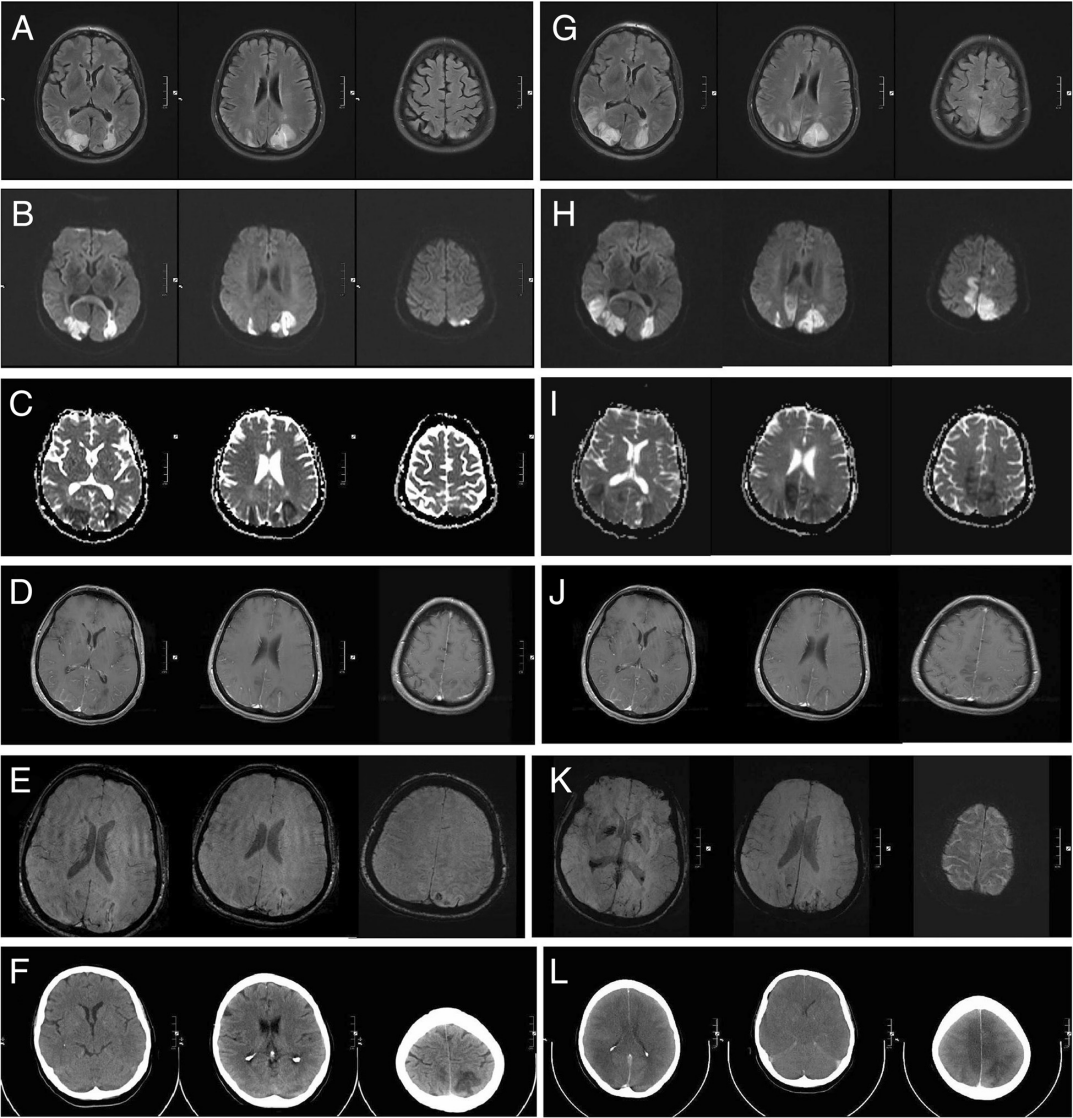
Serial magnetic resonance imaging (3 Tesla) and computed tomography scans showing progressive bilateral reversible posterior leukoencephalopathy syndrome. (A–E) Magnetic resonance imaging immediately after the patient was admitted showed (A) marked hyperintensity in T2 and fluid-attenuated inversion recovery (FLAIR) sequences of the posterior lesions. Diffusion-weighted imaging exhibited restricted diffusion (B) with a decreased signal on apparent diffusion coefficient mapping (C) consistent with cytotoxic edema. Lesions showed slight contrast enhancement (D). Susceptibility-weighted imaging (SWI) showed signal loss indicating the beginning of hemorrhagic lesion transformation (E). (F–J) Magnetic resonance imaging two days after the patient was admitted showed progressive confluent lesions in T2 and FLAIR sequences (F). Increasing areas of restricted diffusion (G) and volume expansion (H) indicated progressive cytotoxic edema. Lesions were still slightly contrast enhancing (I). SWI showed progressive signal loss indicating advancing hemorrhagic lesion transformation (J). (K) A computed tomography scan immediately after admission demonstrates well-demarcated bilateral hypodensities in posterior brain regions. (L) The computed tomography scan on day 3 after admission reveals global cerebral swelling, subsequent upper and lower brainstem herniation and brainstem compression as well as generalized thrombosis of the cerebral sinus and veins.
